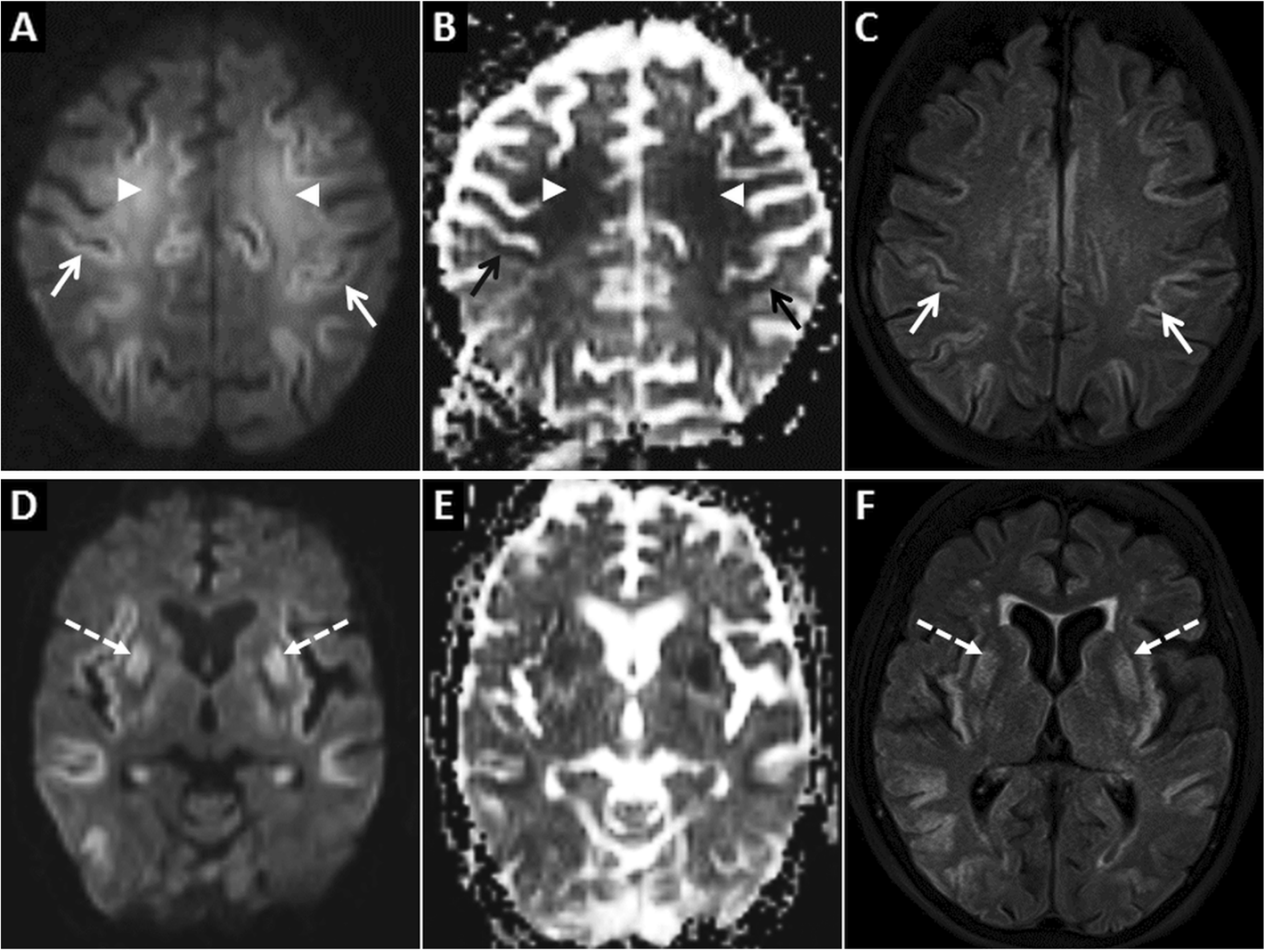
MRI of the brain in a 68-year-old male patient presenting with severe hypoglycaemia. Extensive symmetric GRD (arrows) and restricted diffusion in deep white matter (arrowheads) is seen on axial DWI (a) and ADC image (b). Restricted diffusion is also seen in the putamina bilaterally (dashed arrow) on axial DWI (d) and ADC image (e). Axial FLAIR images (c, f) show hyperintense signal in the involved cortex (arrows) and in the putamina (dashed arrows)
